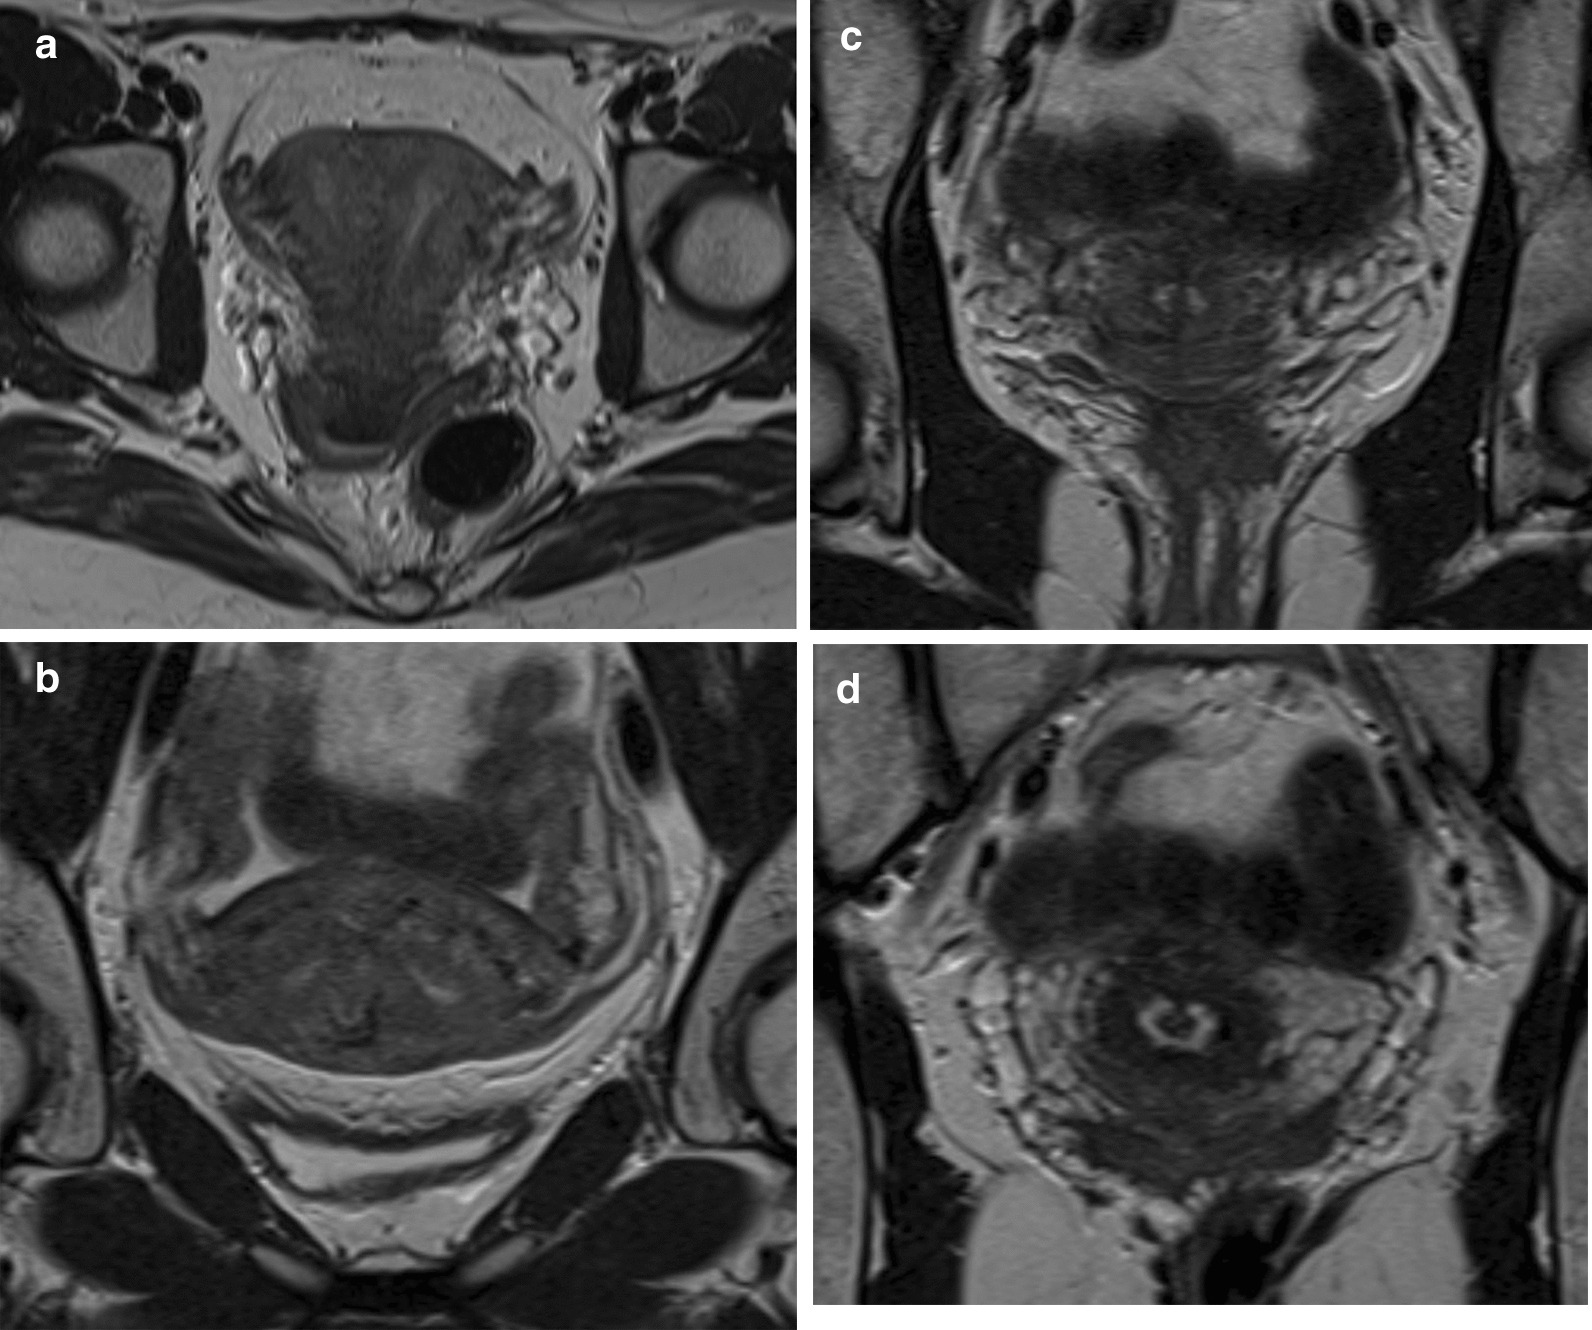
Magnetic resonance imaging at 8 weeks postpartum; a Bicornuate bicollis uterus (functional unicollis) in the transverse plane with both uterine cavities (transverse T2-weighted sequence, Avanto 1.5 T, Siemens Healthineers). b Bicornuate bicollis uterus (functional unicollis): cross-section through both uterine cavities near the fundus (coronary T2-weighted sequence, Avanto 1.5 T Siemens Healthineers). c Bicornuate bicollis uterus (functional unicollis): cross-section through both uterine cavities near the isthmus (coronary T2-weighted sequence, Avanto 1.5 T Siemens Healthineers). d Bicornuate bicollis uterus (functional unicollis): cross-section through the uterine cervix with the postpartum condition of a residual, partly strong cervical septum apically—possibly ruptured peripartum (coronary T2-weighted sequence, Avanto 1.5 T Siemens Healthineers)
